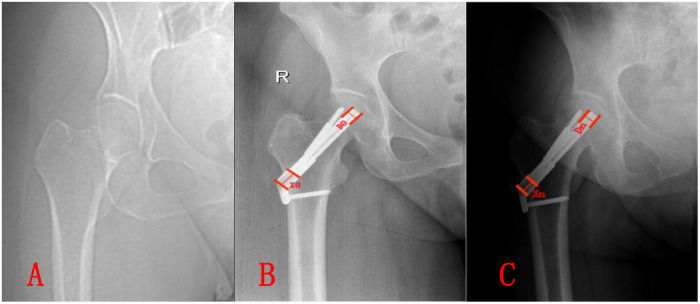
A 43-year-old female patient with a garden type III right femoral neck fracture due to a traffic injury. Open reduction and internal fixation with FNS were performed on the third day after the fracture, following comprehensive examinations. A. Admission x-ray showing a complete fracture, classified as Garden type III femoral neck fracture. B. Immediate postoperative anteroposterior x-ray after FNS fixation, demonstrating satisfactory fracture reduction and centrally positioned FNS internal fixation. C. Follow-up x-ray at 15 months postoperatively, showing blurred fracture lines, acceptable fracture healing, and normal hip function. X₀: distance from the head screw tip to the lateral plate, D₀: bolt diameter, Xₙ, Dₙ: distance from the head screw tip to the lateral plate and bolt diameter measured on the x-ray at 15 months postoperatively, Xᵣ: degree of femoral neck shortening.
